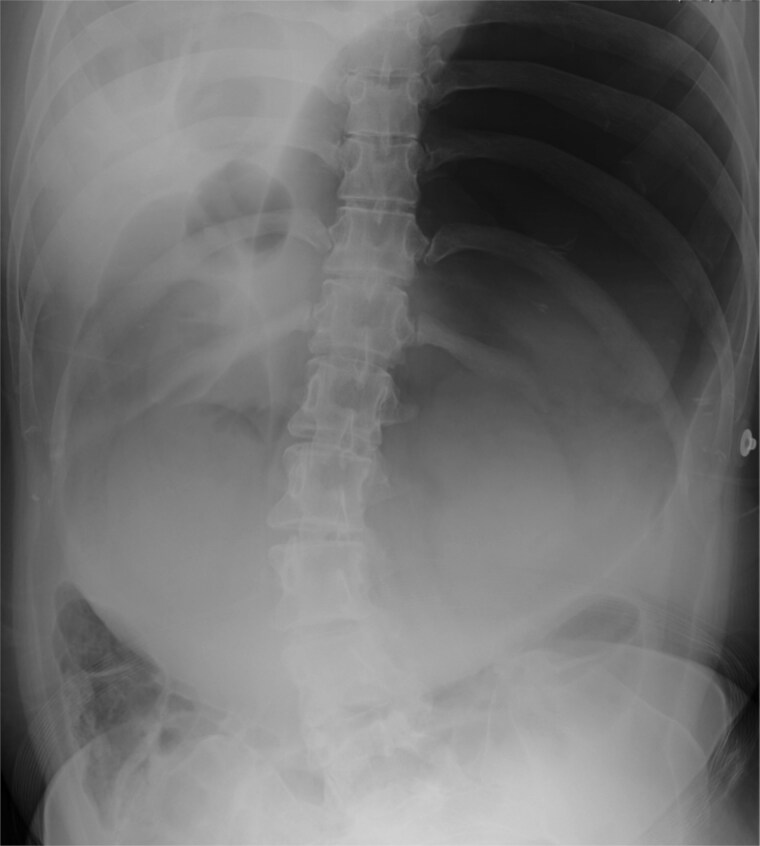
Abdominal radiograph showing marked gastric dilatation, suggesting proximal intestinal obstruction.
